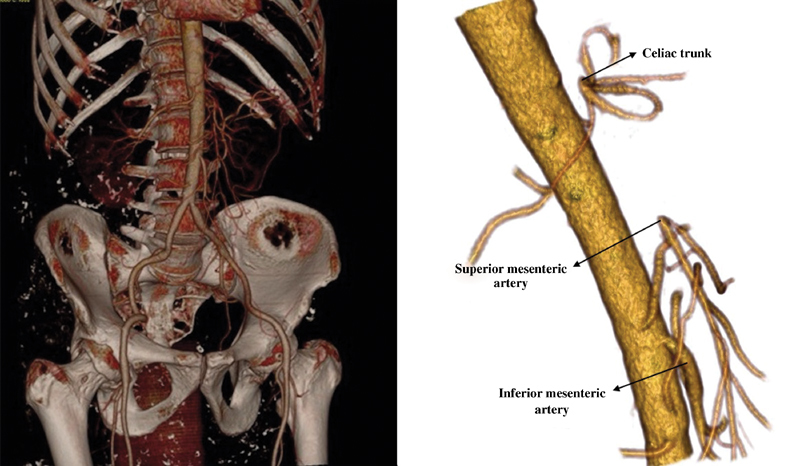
Computed tomography angiography showing occluded celiac trunk and the superior mesenteric artery.
